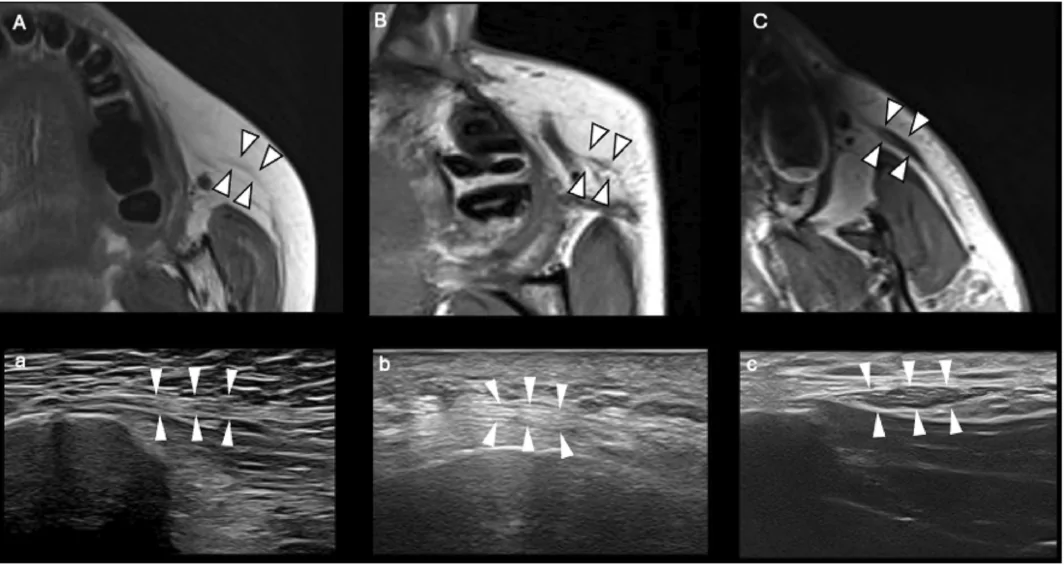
Axial TSE T1‐weighted MRI and transverse high‐frequency US (24–8 MHz) images of the ZMj muscle (black‐lined white arrowhead in MRI imaging and white arrowheads in US imaging) in three study participants. (A and a) In a 26‐year‐old patient, the ZMj shows advanced atrophy with near‐complete fatty replacement (bulk score 3). The muscle appears markedly thinned and nearly isointense to subcutaneous fat on T1‐weighted MRI (A, black‐lined white arrowheads), and thinned and hyperechoic on US (a, white arrowheads). (B and b) In a 59‐year‐old patient, moderate atrophic changes are present, with partial fatty infiltration (bulk score 2). The ZMj muscle remains partially hypointense on T1‐weighted MRI (B, black‐lined white arrowheads) and appears thinned and hyperechoic on US (b, white arrowheads). (C and c) In a 61‐year‐old patient, the ZMj shows preserved morphology and signal characteristics (bulk score 1), appearing thick and hypointense relative to subcutaneous tissue on T1‐weighted MRI (C, black‐lined white arrowheads), and hypoechoic on US (c, white arrowheads). TSE, turbo spin‐echo; MRI, magnetic resonance imaging; US, ultrasound; ZMj, zygomaticus major.
